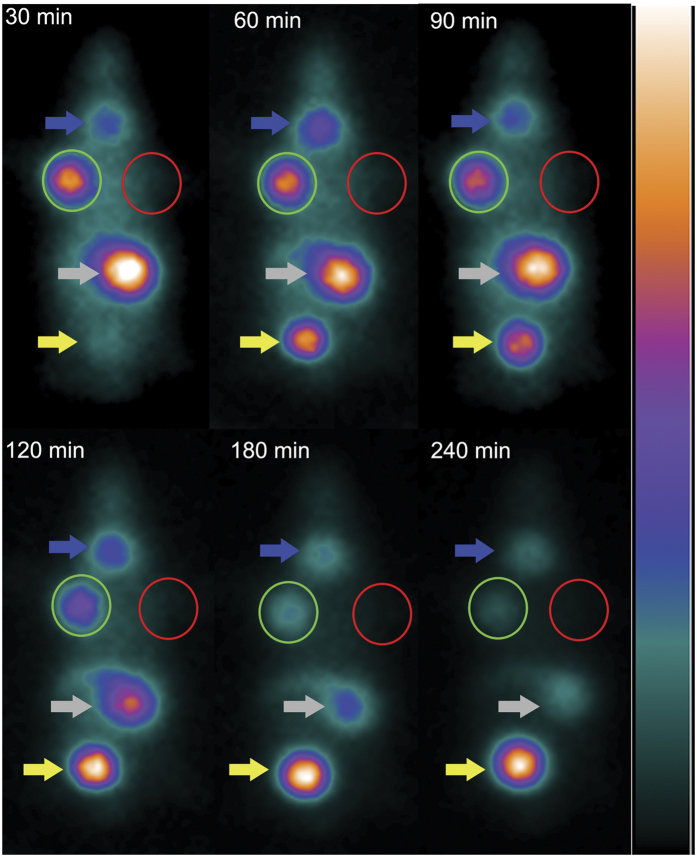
The dynamic imaging by SPECT instrument. The imaging variation over time in the first SPECT imaging (20^th^ day after transplantation with screened Bac-NIS-SB-NeoR-U87 cells). The green and red circle respectively represent the Bac-NIS-SB-NeoR-U87 cells and U87 cells. Blue, gray and yellow arrows respectively represent thyroid, stomach and bladder.
